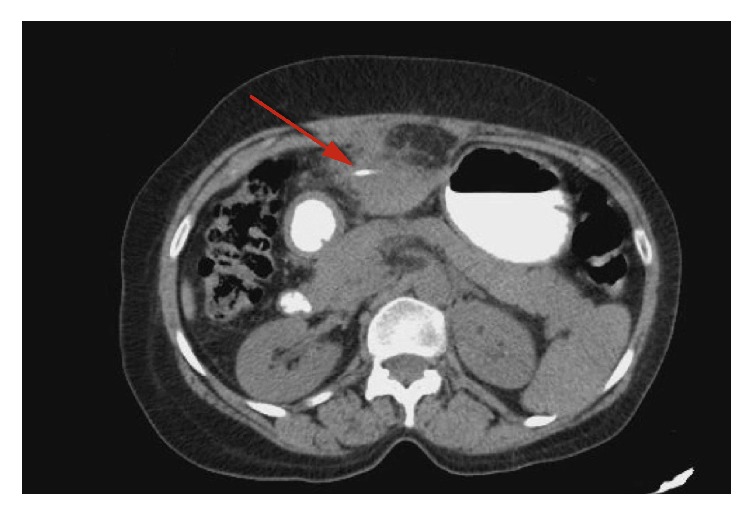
Axial view CT scan of the abdomen and pelvis showing a radiopaque FB in the liver (red arrow).
